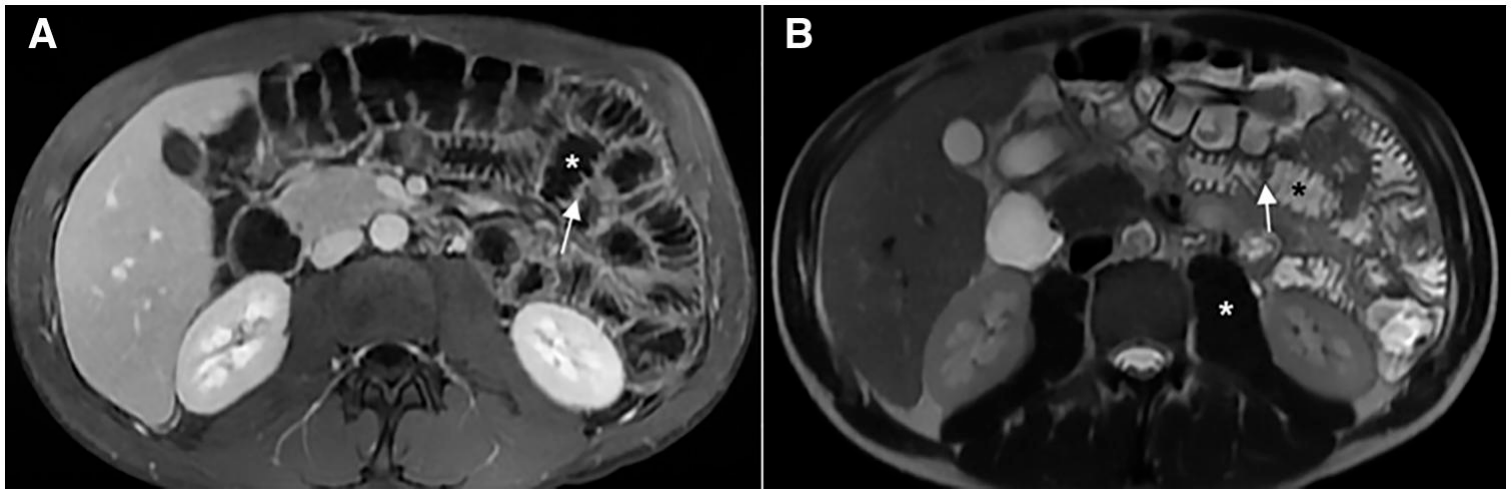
Example of small bowel distension by oral biphasic contrast medium (polyetilen-glycole solution) in MR-enterography. (A) Axial T1-weighted image after gadolinium injection: lumen of jejunal loops is well distended and it shows low signal (white asterisk); the bowel wall (white arrow) shows homogeneous thin enhancement and it is clearly distinguished from lumen. (B) Axial T2-weighted image shows hyperintense signal of lumen of jejunal loops (black asterisk); the bowel wall (white arrow) has an intermediate signal, lower than the lumen (black asterisk) and higher than the psoas muscle (white asterisk).
